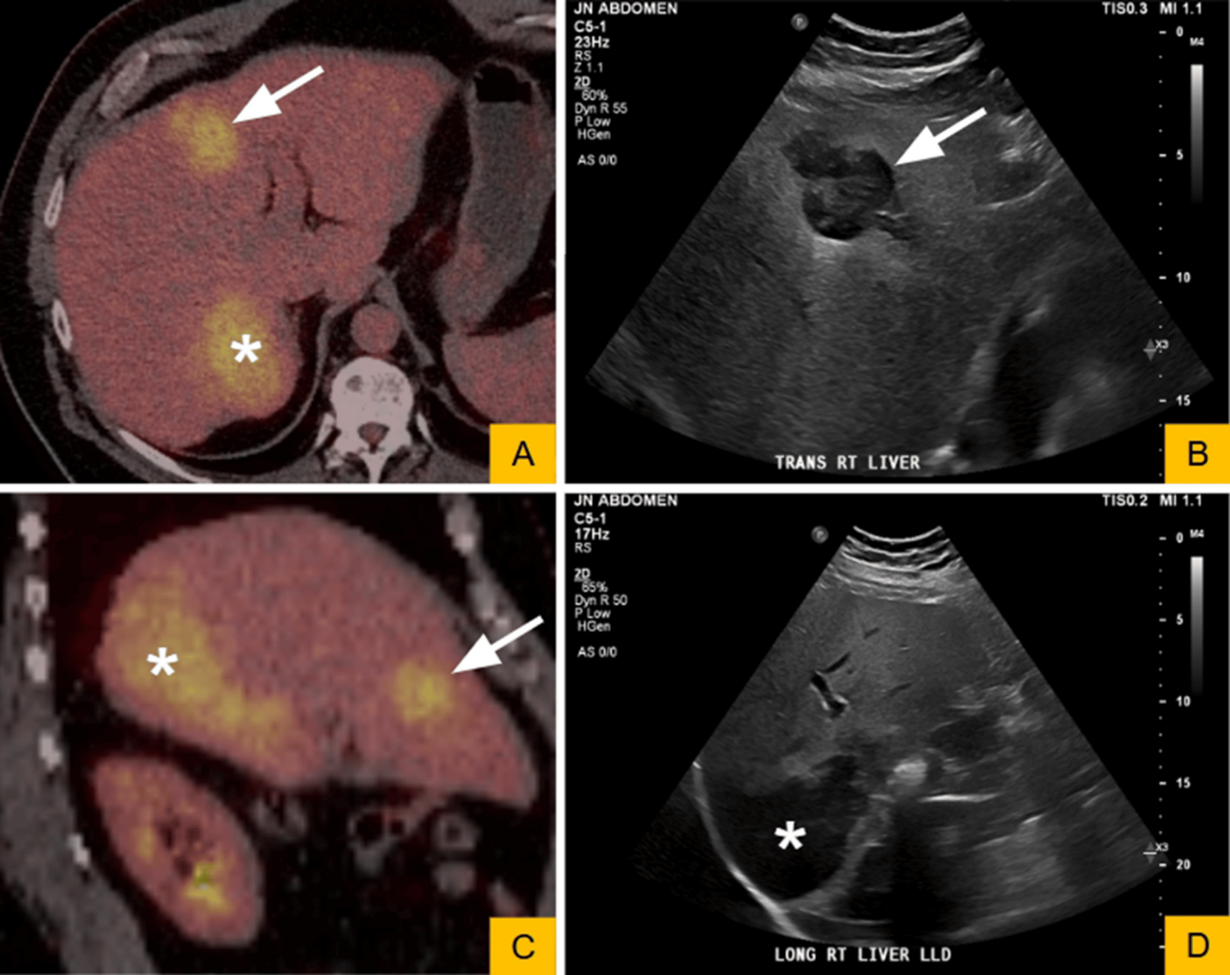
Imaging findings of hepatic involvement by plasma cell leukemia. (A) Axial and (C) sagittal fused fluorodeoxyglucose positron emission tomography (FDG-PET)/non-contrast CT images demonstrate two FDG-avid hepatic masses involving segments 4A/B (arrows) and 7 (asterisks). The segment 7 lesion shows a posterior exophytic component. (B) Corresponding transverse and (D) longitudinal grayscale ultrasound images demonstrate well-circumscribed hypoechoic masses. The anterior lesion in segment 4A/B (arrows) measures up to 5.2 cm, and the posterior segment 7 lesion (asterisks) measures up to 12.2 cm.
